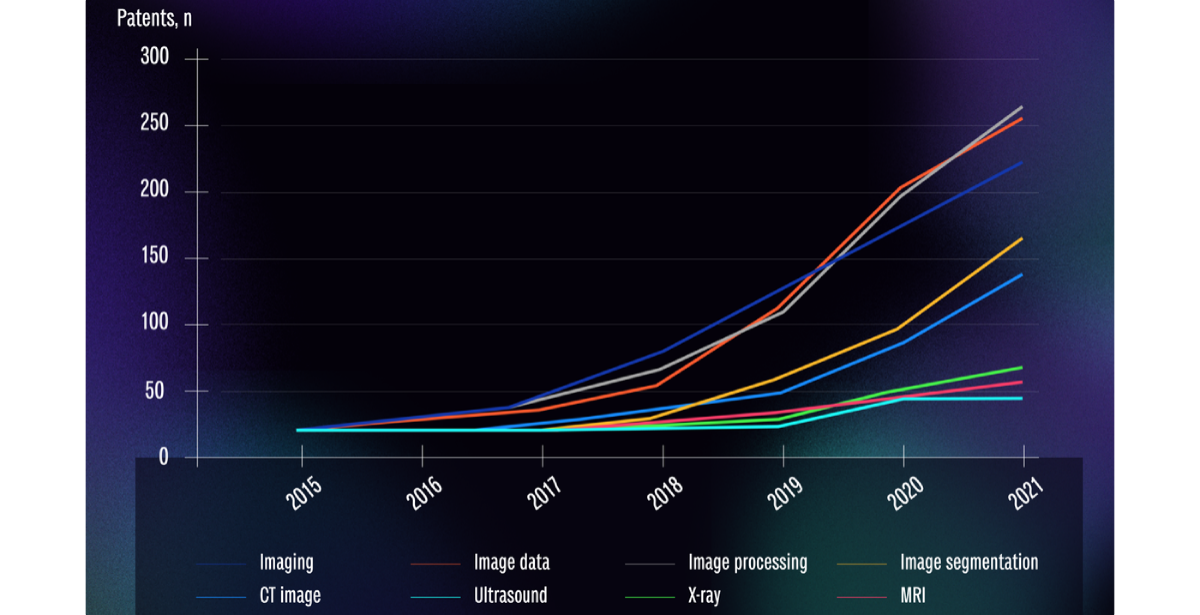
Trends in imaging-based patents. CT: computed tomography; MRI: magnetic resonance imaging.
